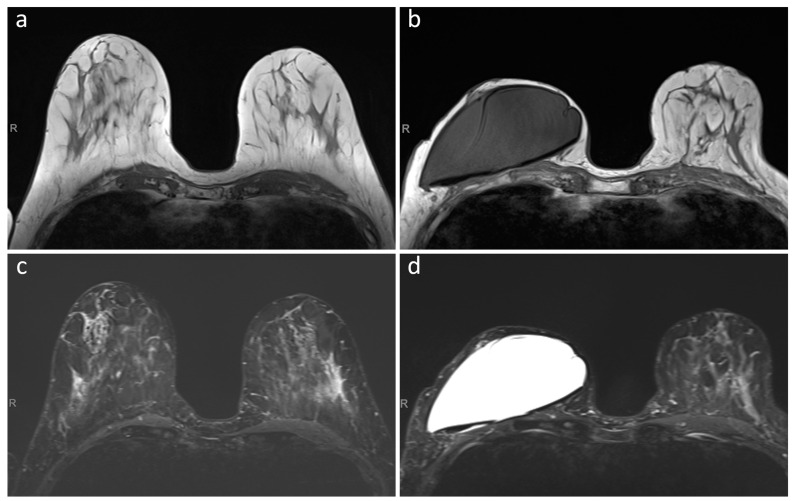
T1-weighted (panels (a,b)) and T2-weighted (panels (c,d)) MRI images of a patient that underwent a skin sparing mastectomy of the right breast with pre-pectoral implantation of the MRI-conditional breast tissue expander. (Panels (a,c)) show preoperative MRI images, while (panels (b,d)) show MRI images acquired prior to planned exchange surgery to definitive implant, with no negative effects on image quality caused by the presence of the tissue expander and its non-metallic port.
